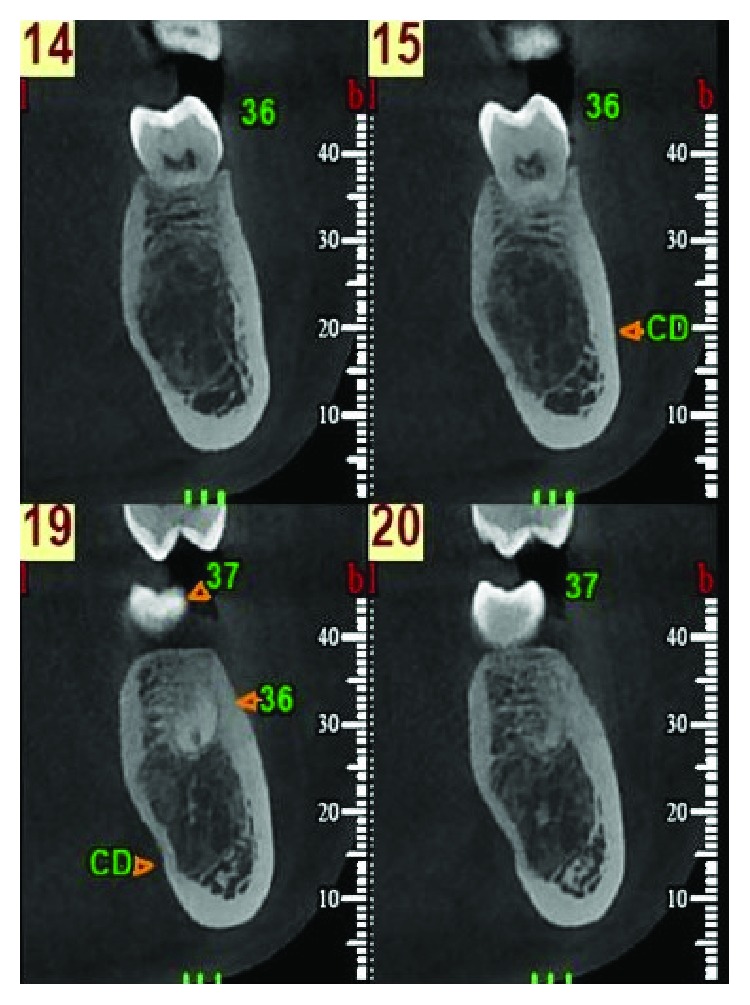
Cone beam computerized tomography within 18 months of canalization. Complete replacement of the radiolucent image is observed, with a density compatible with normal bone tissue in the process of calcification. The lingual cortical plate adjacent to the lesion in the area of teeth 35-37 presents normal thickness.
